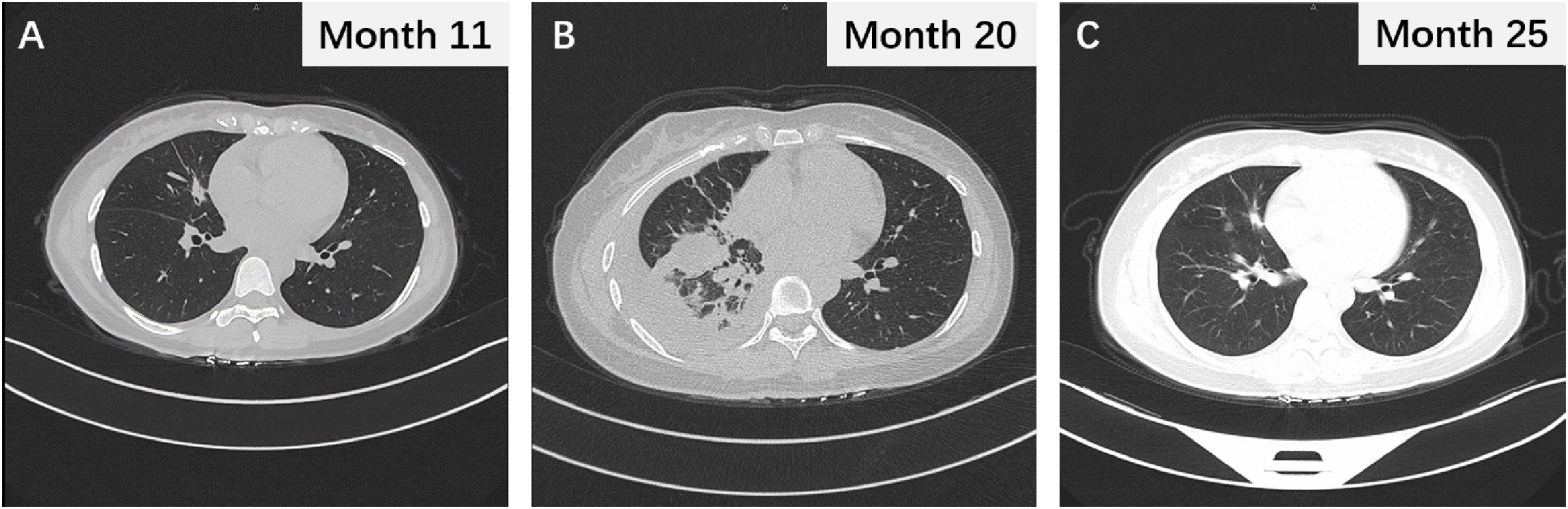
(A) The thoracic CT scan revealing the changes in lung nodules after 2 months of osimertinib treatment. (B) The chest CT image following termination of pregnancy. (C) Chest CT scan after 16 months of osimertinib treatment and demonstrating sustained partial response.
